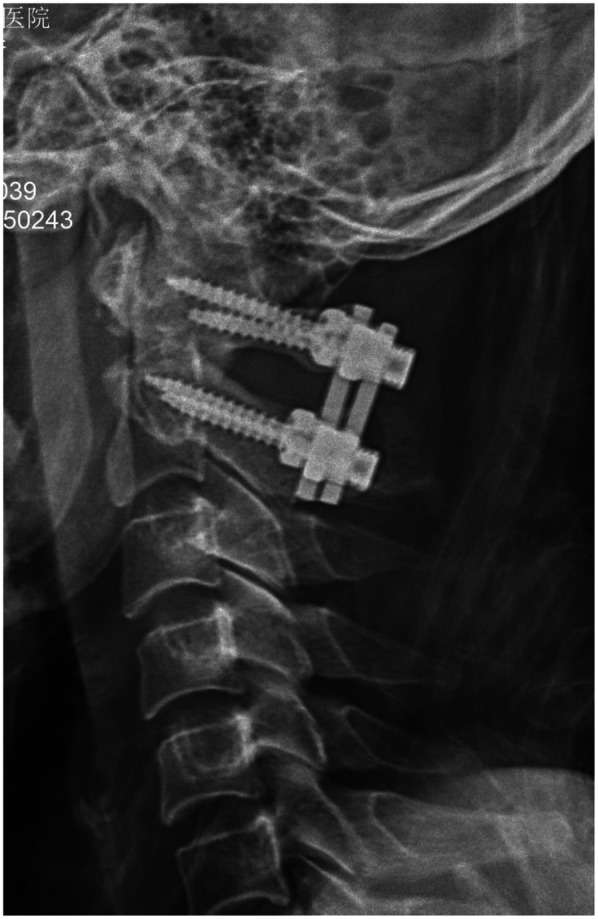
Lateral x-ray after pedicle screw fixation surgery. Postoperative x-ray showed fracture reduction.
